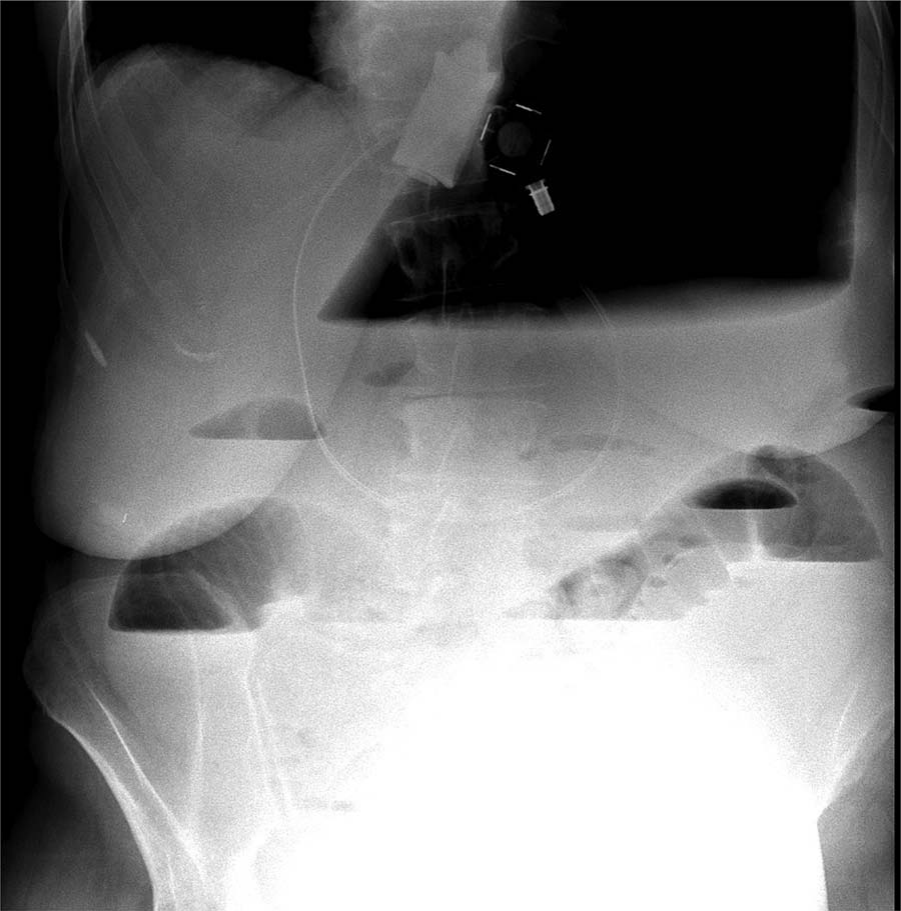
X-ray massive gastric dilatation and small bowel dilatation with air-fluid levels.
